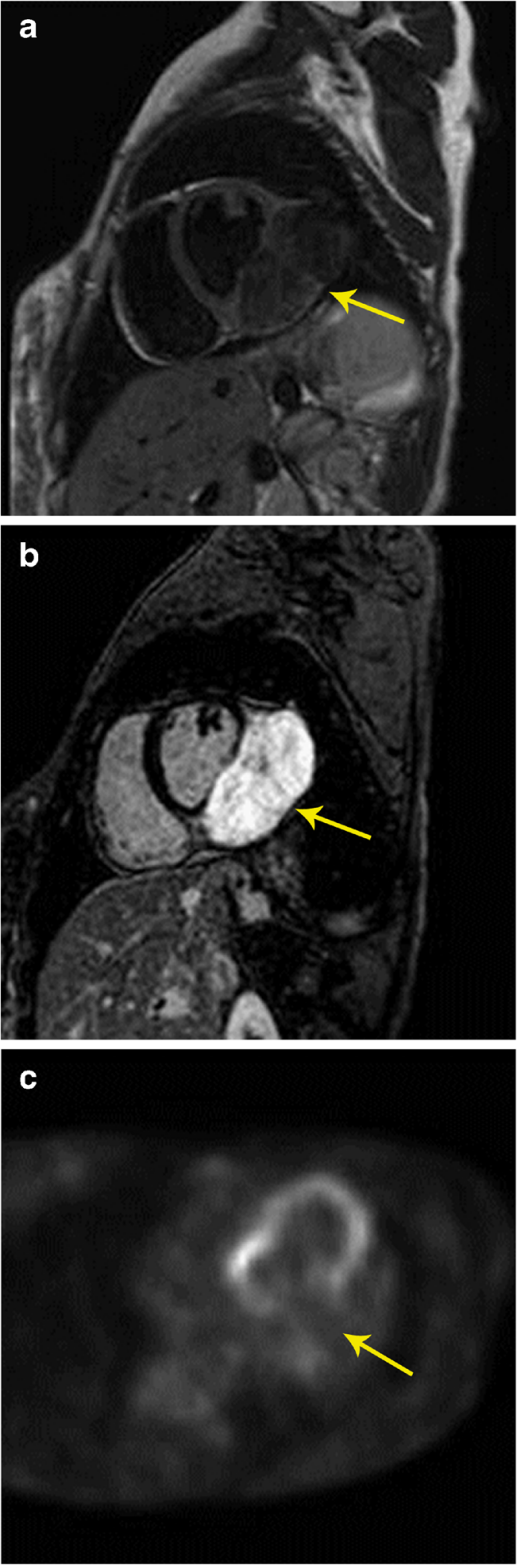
Characterization of mass. a 20-year-old female with irregular heart rate noted on physical exam, which eventually led to a cardiac MRI. Short-axis T2-weighted image shows intermediate to low signal 9 × 4 cm mass originating from the inferolateral basal to mid left ventricle (arrow). b There was no immediate contrast enhancement (not shown here), but there is intense delayed contrast enhancement in short-axis inversion recovery sequence (arrow). c Four-chamber FDG-PET scan shows no abnormal uptake in the mass, indicating a benign mass. The imaging findings were thought to be suggestive of fibroma. Based upon these findings, and the patient’s lack of symptoms, a choice was made to not intervene, and instead to obtain clinical follow-up as well as serial imaging. The patient has done well over six years, and the mass has shown no interval change in size
